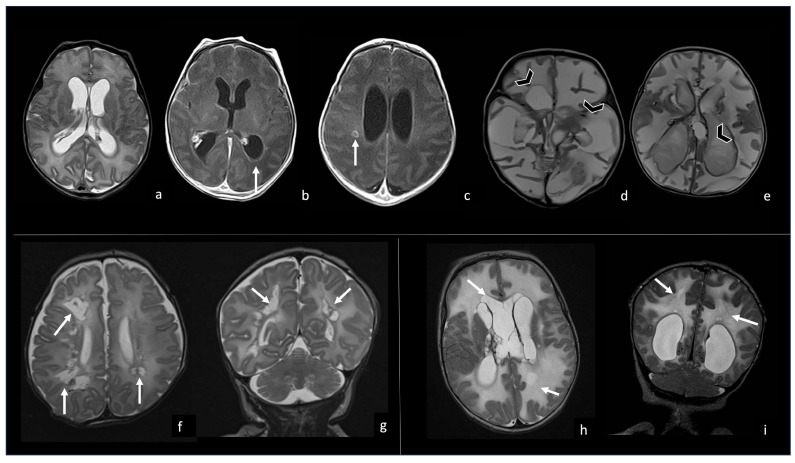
Three different cases of newborns with brain sequelae caused by *Listeria Monocytogenes* meningoencephalitis. The first case (a–e) refers to a boy of 9 days of life, who presented with meningoencephalitis characterized by ventriculitis with enlarged ventricles ((a), axial T2WI) with ependymal contrast enhancement (arrow in (b), axial post-contrast T1WI) and an encapsulated abscess in the right white matter (arrow in (c), axial post-contrast T1WI). At the 3-month FU, the brain MRI showed massive cystic encephalomalacia and periventricular cavitations (arrowheads in (d,e), axial T2WI). The second case (f,g) shows the 3-month FU MRI of a girl affected by listeria meningoencephalitis at 6 days of life, evolved in multiple periventricular cysts (arrows in (f), axial and (g), coronal T2WI). The third case (h,i) refers to a boy who presented with *Listeria* meningoencephalitis at 5 days of life, whose 2-month FU MRI showed encephalomalacia with multiple cysts (arrow in (h), axial and (i), coronal T2WI).
